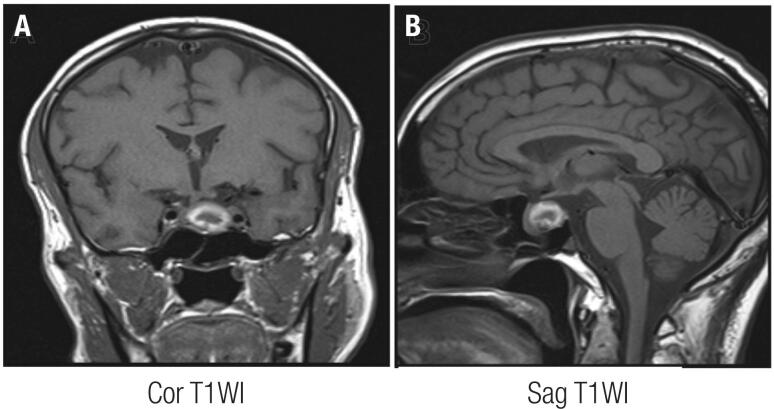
Coronal (A) and sagittal T1-weighted imaging (B) sequences of magnetic resonance imaging performed in a patient with classical pituitary apoplexy, 30 days after the acute event and showing a ring area of hyperintense signals in the sellar lesion.
